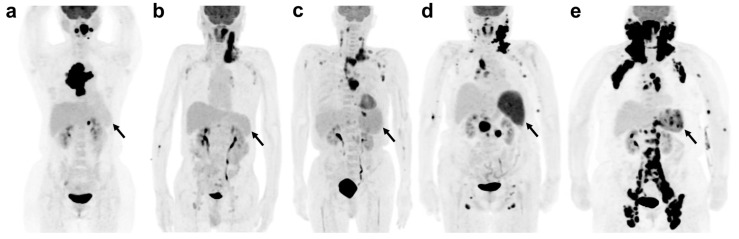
Representative cases of splenic [^18^F]FDG uptake patterns on baseline PET/CT in DLBCL. Maximum intensity projection images from initial staging [^18^F]FDG PET/CT. (a) A 40-year-old woman shows negative splenic uptake lower than hepatic uptake (Deauville score 2); the patient remained relapse-free during follow-up. (b) A 70-year-old man exhibits positive diffuse splenic uptake higher than the mediastinal blood pool but lower than or equal to the liver (Deauville score 3). (c) A 70-year-old man demonstrates positive diffuse splenic uptake moderately higher than hepatic uptake (Deauville score 4). (d) An 85-year-old woman shows splenomegaly with positive diffuse splenic uptake markedly exceeding hepatic uptake (Deauville score 5). (e) A 67-year-old woman shows positive focal splenic uptake superimposed on diffuse splenic uptake. All four patients with positive splenic uptake patterns (b–e) experienced disease relapse. Arrows indicate the spleen demonstrating the described uptake pattern.
